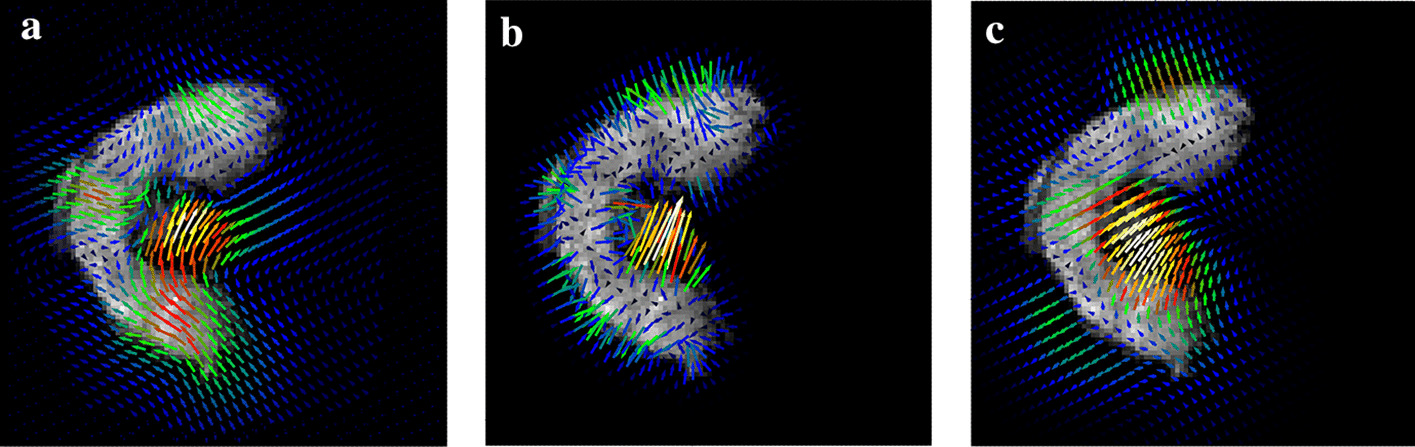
Vector fields obtained by deformable registration methods. The vectors correspond to the displacements applied to the MS image to match the MR image, obtained by : a the variational method of Modersitzki [21] or free form deformation models with two different parameters b
